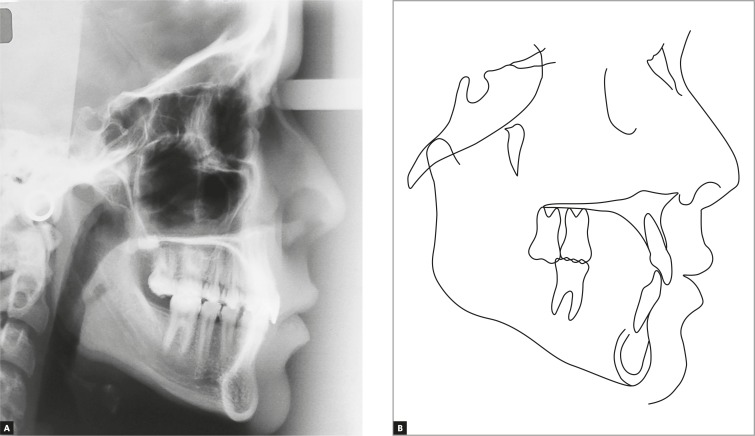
Initial profile cephalometric radiograph (A) and cephalometric tracing
